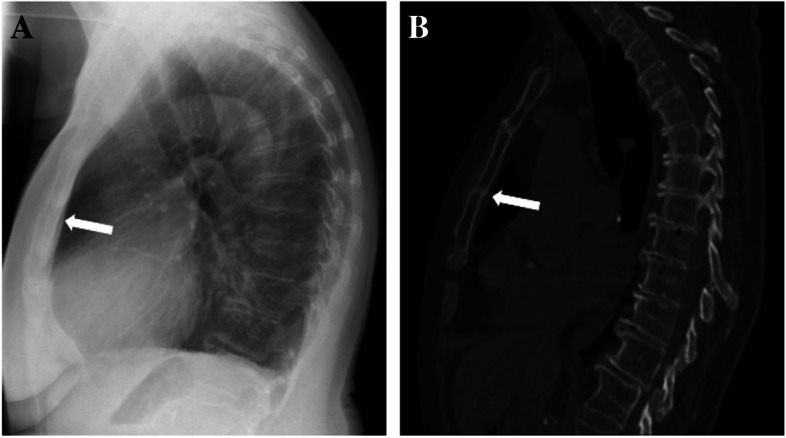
Isolated sternal fracture in a lateral chest x-ray (A) and a sagittal chest computed tomography view (B)
